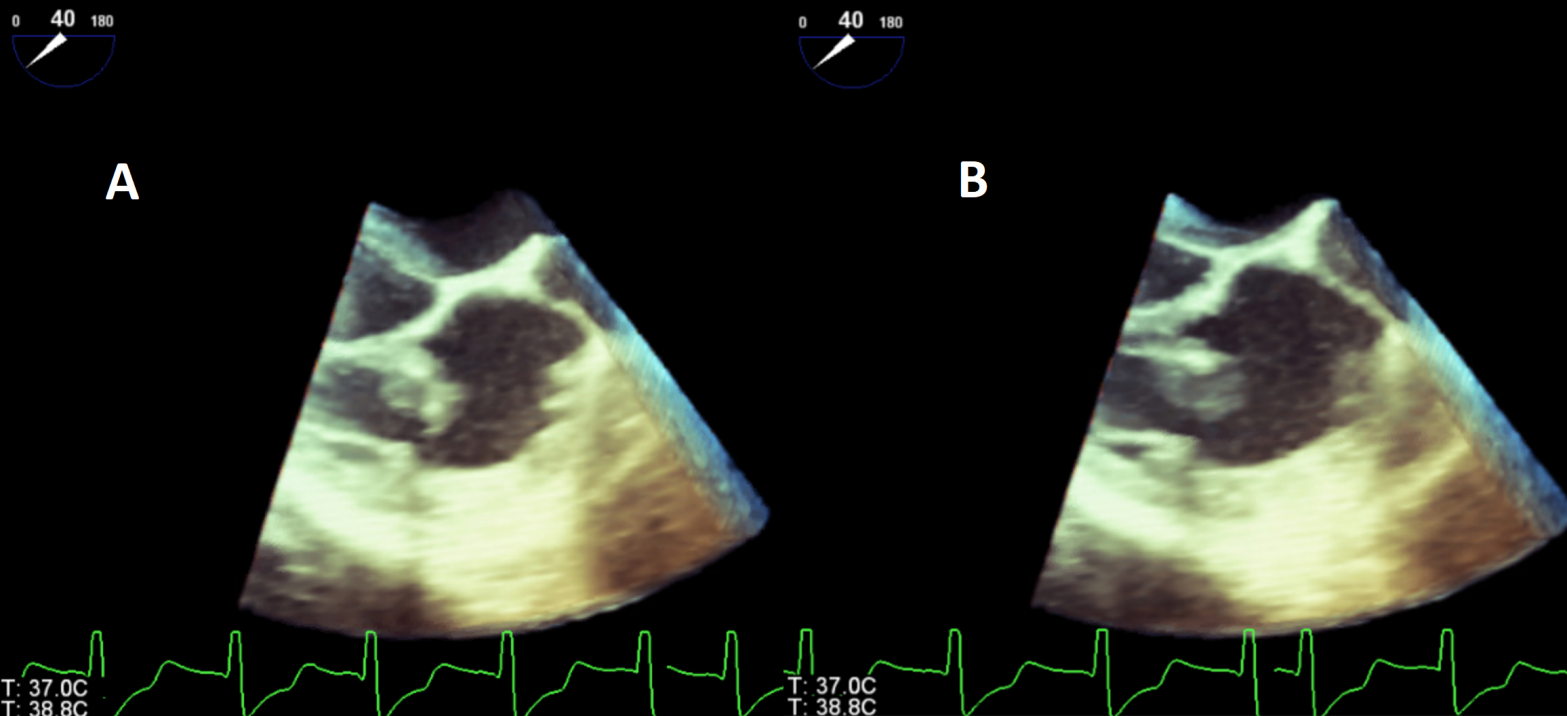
(A–B) Transesophageal echocardiogram at mid-esophagus showing
